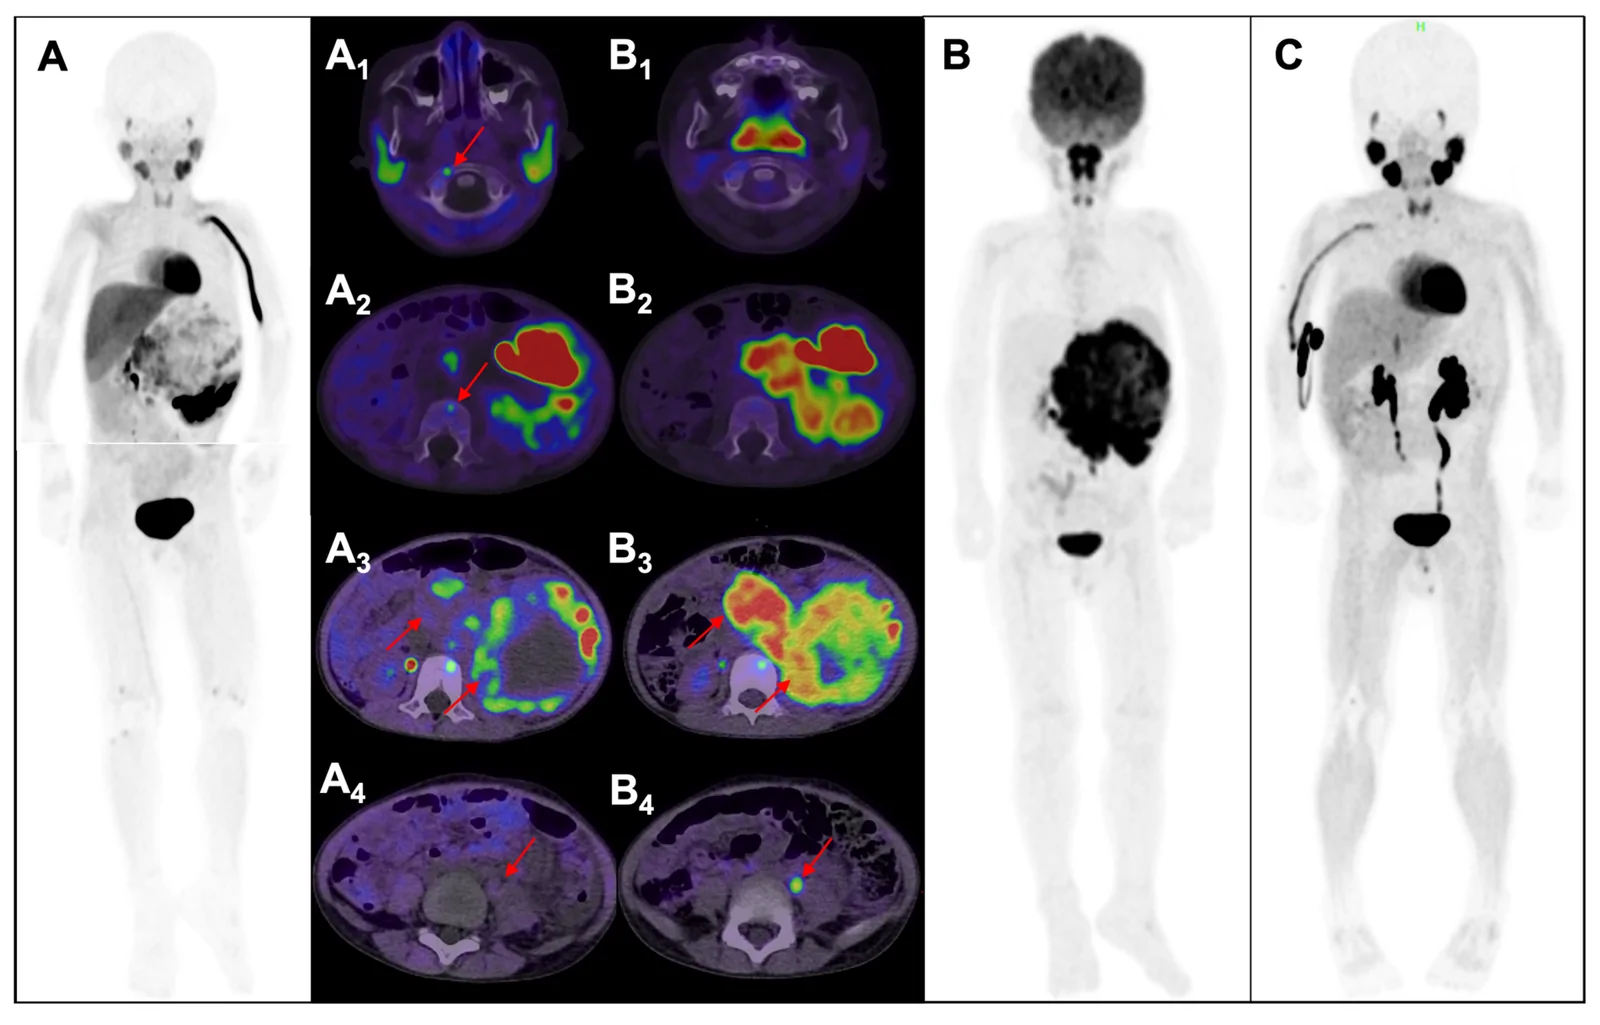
[^18^F]MFBG PET showing bone marrow involvement not detected by [^18^F]FDG PET in a pediatric patient with newly diagnosed neuroblastoma. A 4-year-old boy presenting with fever and abdominal pain. Anatomic imaging (abdominal CTA and abdominopelvic MRI) revealed a large retroperitoneal mass suggestive of neuroblastoma. [^18^F]MFBG PET/CT MIP (A) and axial PET/CT fusion images demonstrated MFBG-avid lesions in the C1 vertebra (A₁) and L2 vertebra (A₂), as well as heterogeneous MFBG uptake within the primary tumor (A₃). However, an enlarged pelvic lymph node shows no appreciable MFBG uptake, consistent with a false-negative lesion (A₄). [^18^F]FDG PET/CT MIP (B) and corresponding axial fusion images show the absence of FDG uptake in C1 (B₁) and L2 (B₂), positive FDG uptake in the primary tumor and lymph node lesions (B₃ and B_4_). Follow-up [^18^F]MFBG PET/MR (C) after therapy shows no residual abnormal uptake.
